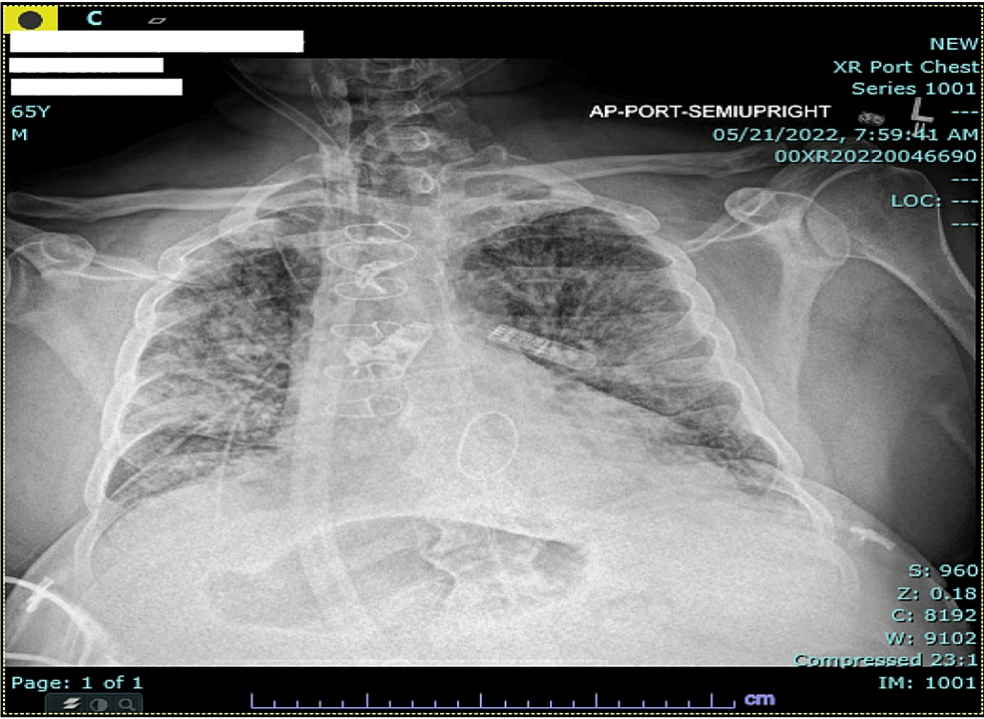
Portable chest X-ray prior to ICU transfer The image shows worsening fluffy airspace opacifications of bilateral lungs compatible with acute respiratory distress syndrome (ARDS)
